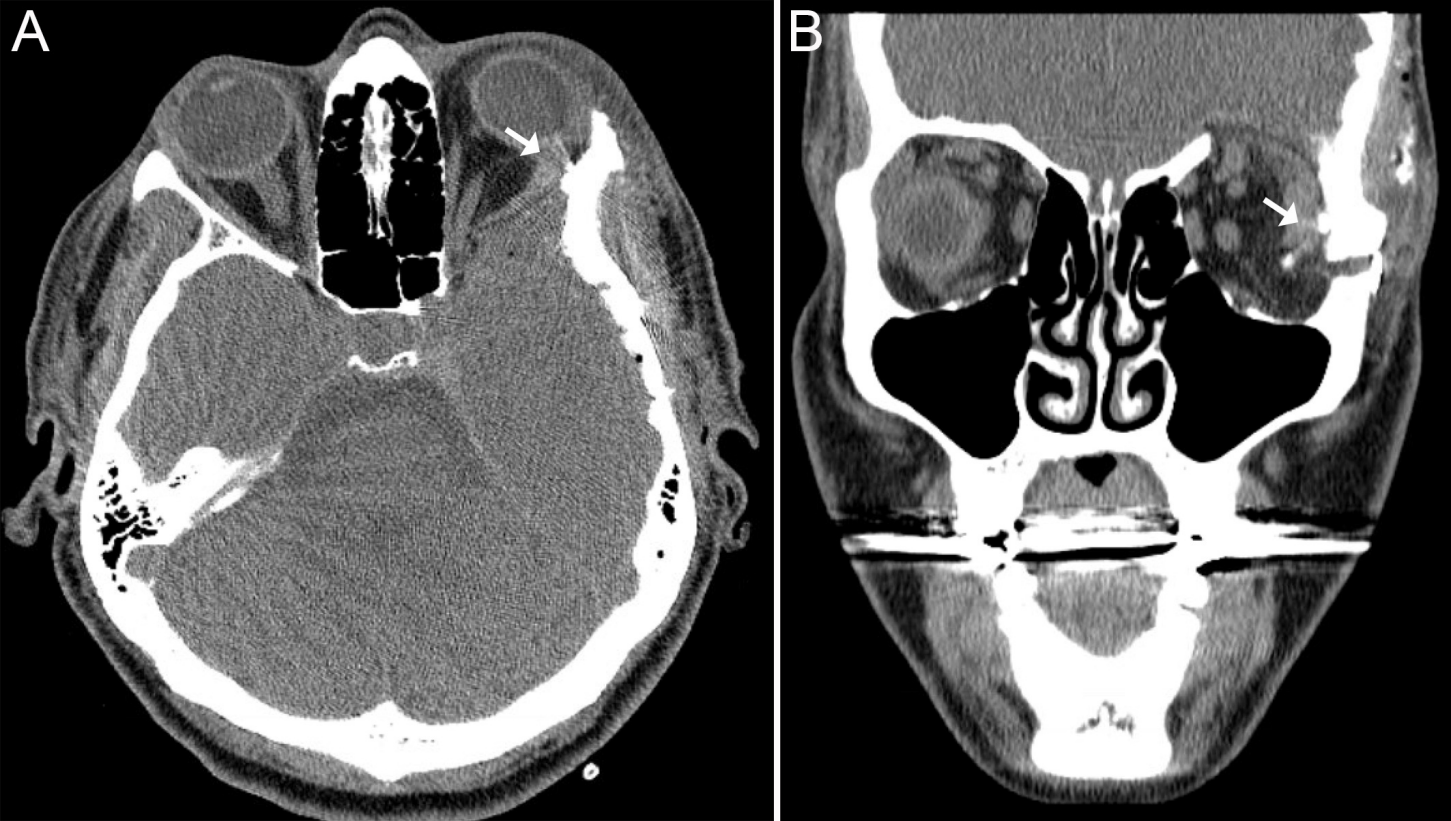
Computed tomography of the orbits in Case 2 Computed tomography of the orbits in Case 2 demonstrates impingement of the left lateral rectus muscle between the residual zygomatic bone and alloplastic implant on axial image (A, arrow) and coronal image (B, arrow). The axial image highlights the abnormal course of the muscle.
